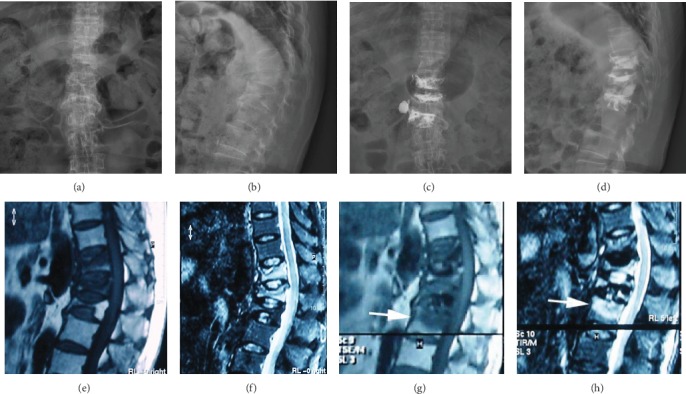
Preoperative X-ray of a 69-year-old woman with OVCFs of the T11, 12, and L1 vertebral bodies (a, b). MRI (T1-weighted and STIR-weighted images) visualized each fractured vertebral body (e, f). The BMD values were -3.5 (T11), -3.3 (T12), and -3.6 (L1). The patient underwent PKP from T11 to L1 (c, d). Seventeen days after surgery, the patient complained of low back pain, and MRI showed a new vertebral body fracture in L2 (white arrow) (g, h). The patient elected to receive conservative treatment.
